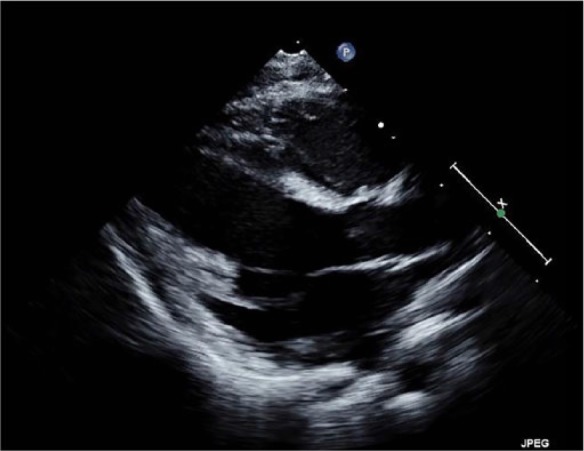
Transthoracic echocardiogram (parasternal long axis view at end-diastole), prior to pocapavir treatment, demonstrated a hyperechoic basal and mid-ventricular septum, papillary muscle, and posterior left ventricular (LV) wall; LV systolic function was severely depressed.
